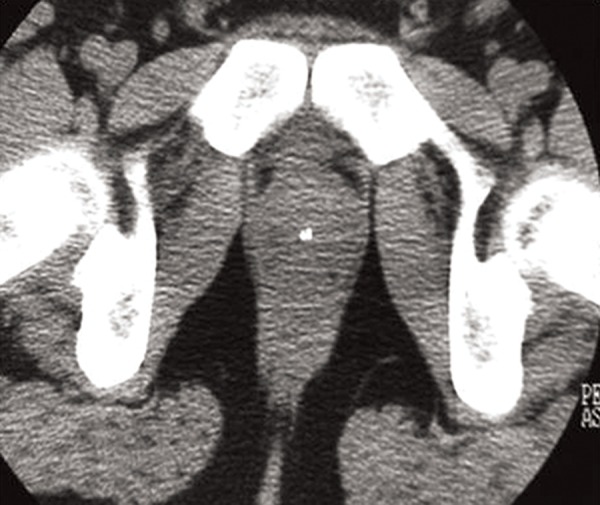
Pelvic HRCT: calcification in the prostate. HRCT; High resolution computerized tomography scan.
